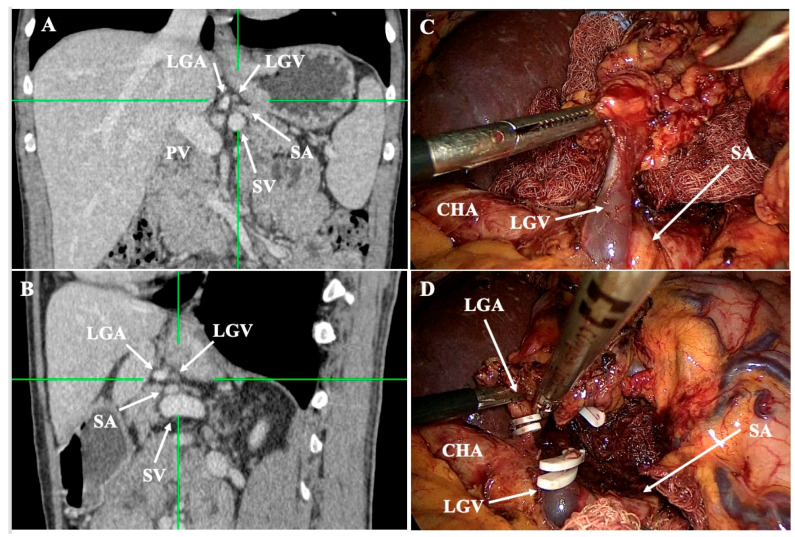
Preoperative computed tomography angiography (CTA) (A,B) and intraoperative images (C,D) showing the LGV coursing anterior to the splenic artery (SA). This configuration (type IV) is associated with higher bleeding risk during suprapancreatic dissection and highlights the value of CTA in mapping LGV variants prior to radical gastrectomy [30]. Personal archive of Assistant Professor Orestis Lyros.
